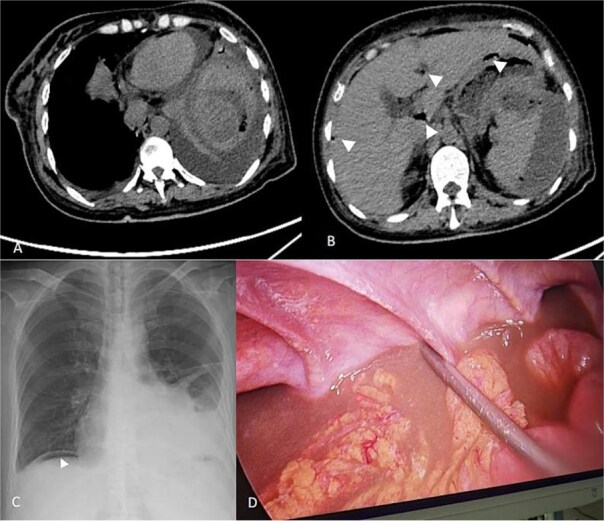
CT scan of spleen abscess (A) and free gas in the abdominal cavity (B) before the surgery and splenic Puncture. Free gas in the abdominal cavity on the chest X-ray (C). The scene during laparoscopic examination (D). Note: The triangle indicates the free gas in the abdominal cavity.
